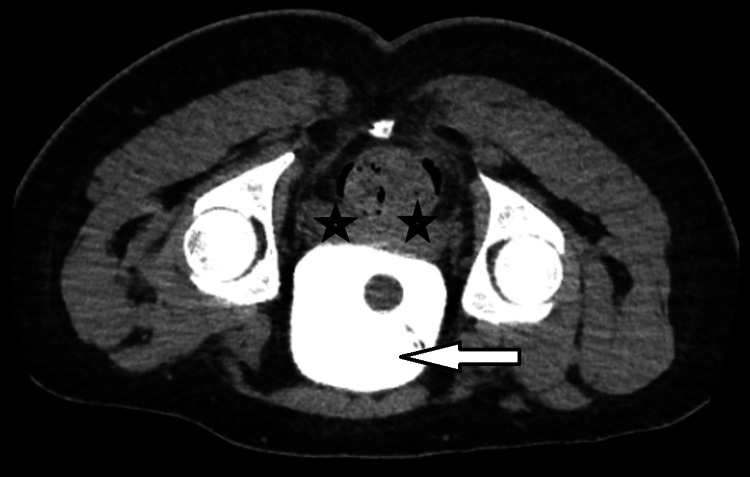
CT cystogram axial view 10 days after surgery (prone position) showing the bladder full of contrast (white arrow) with no contrast leak in the peri-vesical area (black stars). White arrow: urinary bladder with contrast, black stars: peri-vesical area with no contrast leak CT: computerized tomography
